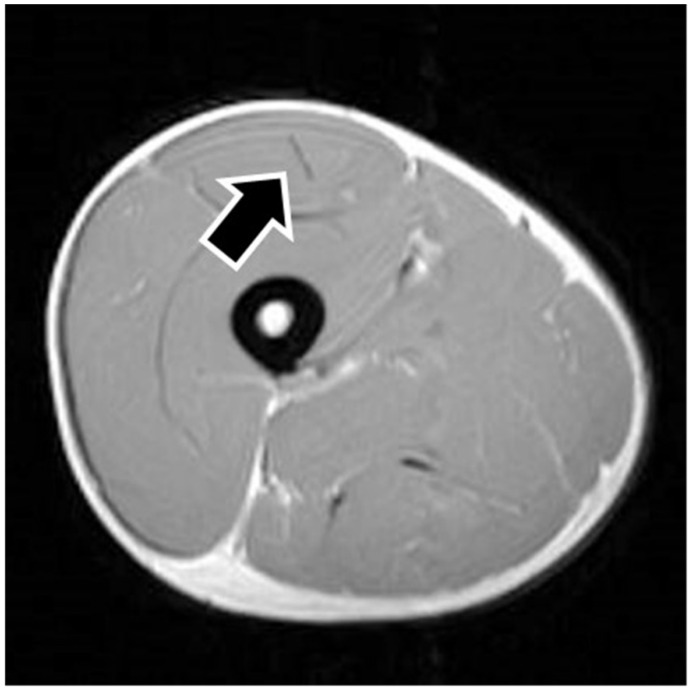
Noninjured rectus femoris muscle. The figure shows an example of noninjured rectus femoris muscle. The axial T1-weighted image does not show a low signal in the central tendon (black arrow).
